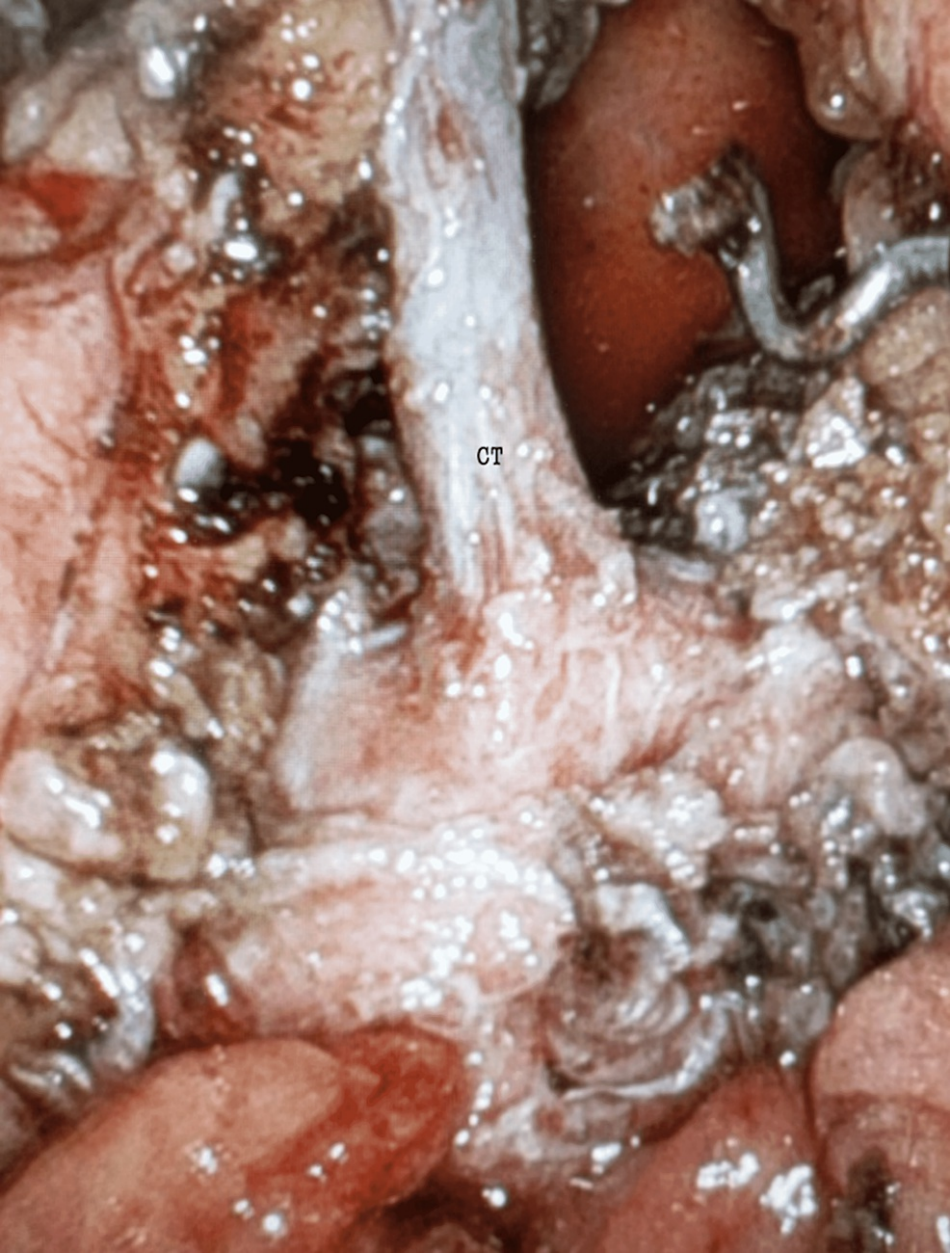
Median arcuate ligament release and celiac neurolysis. CT = celiac trunk
